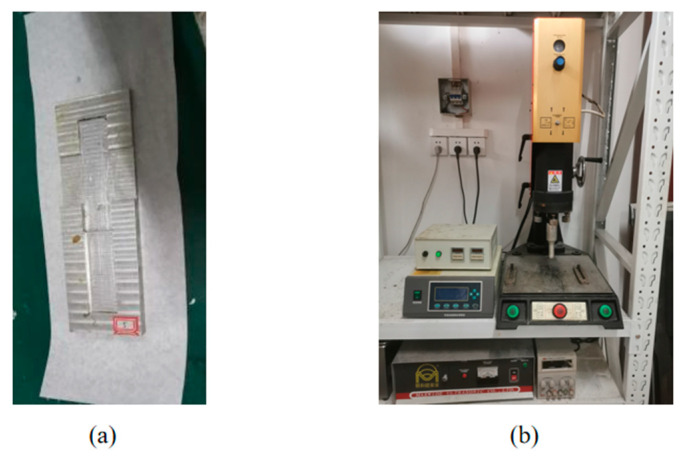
The fixture (a) and ultrasonic workbench (b).
